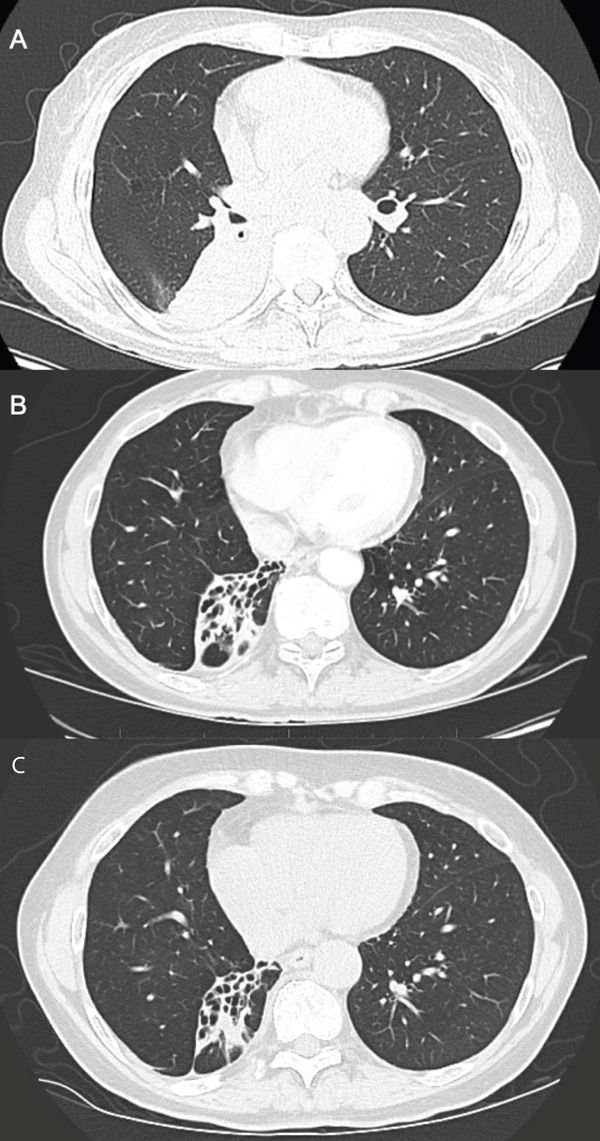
(A) CT chest 2 weeks prebronchoscopy, (B) CT chest 2 months postbronchoscopy, and (C) CT chest 9 months postbronchoscopy.
